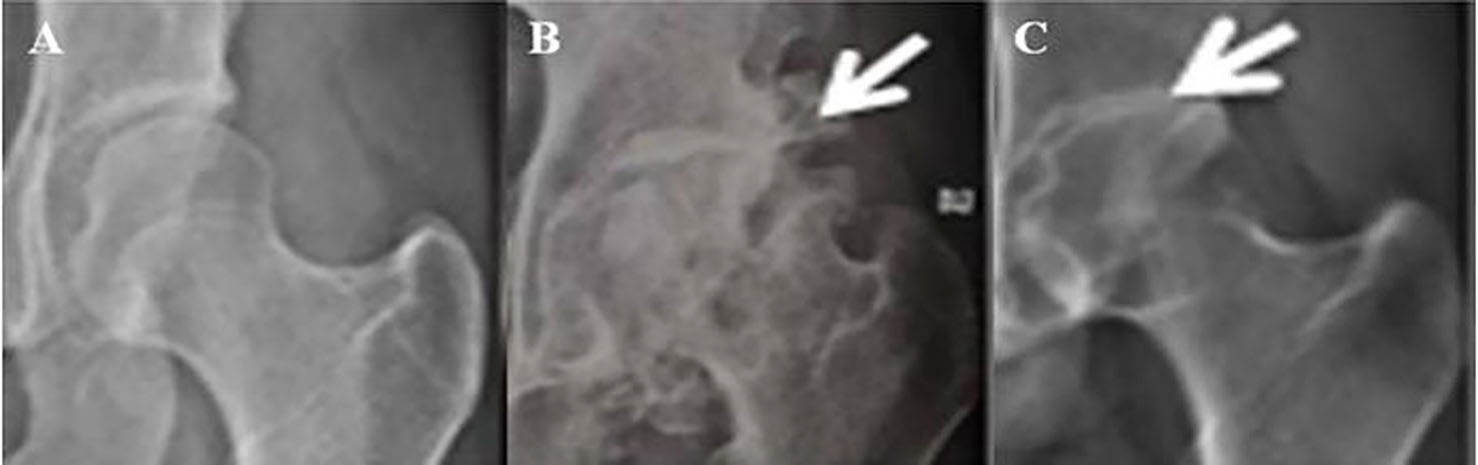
X-rays of hips in a healthy control, OA and AS subject. (A) a healthy control subject; (B) an OA patient. White arrow points at an osteophyte; (C) an AS patients. White arrow points at narrowing of joint space.
